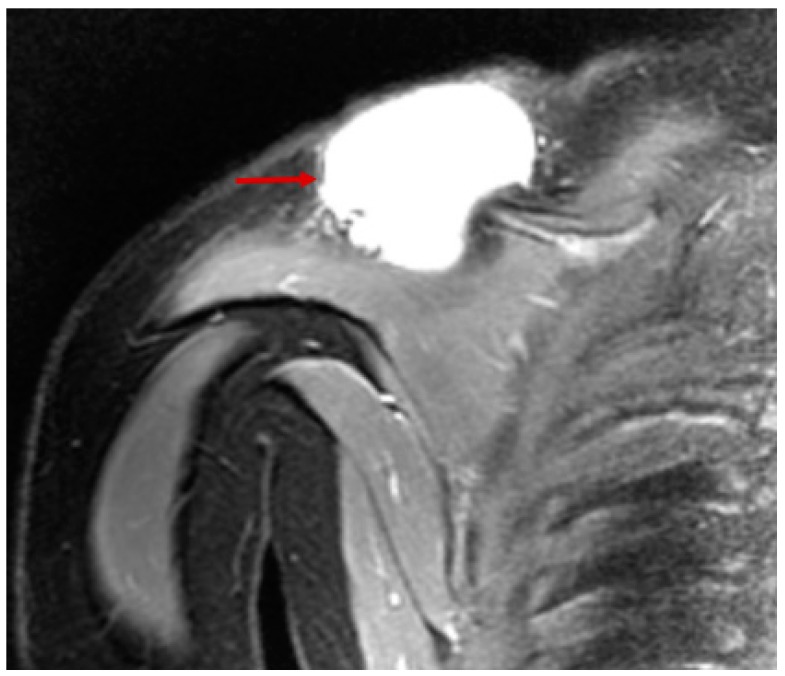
Coronal MRI of extraosseous Ewing sarcoma. Note cystic nature of lesion on T2 weighted image.
